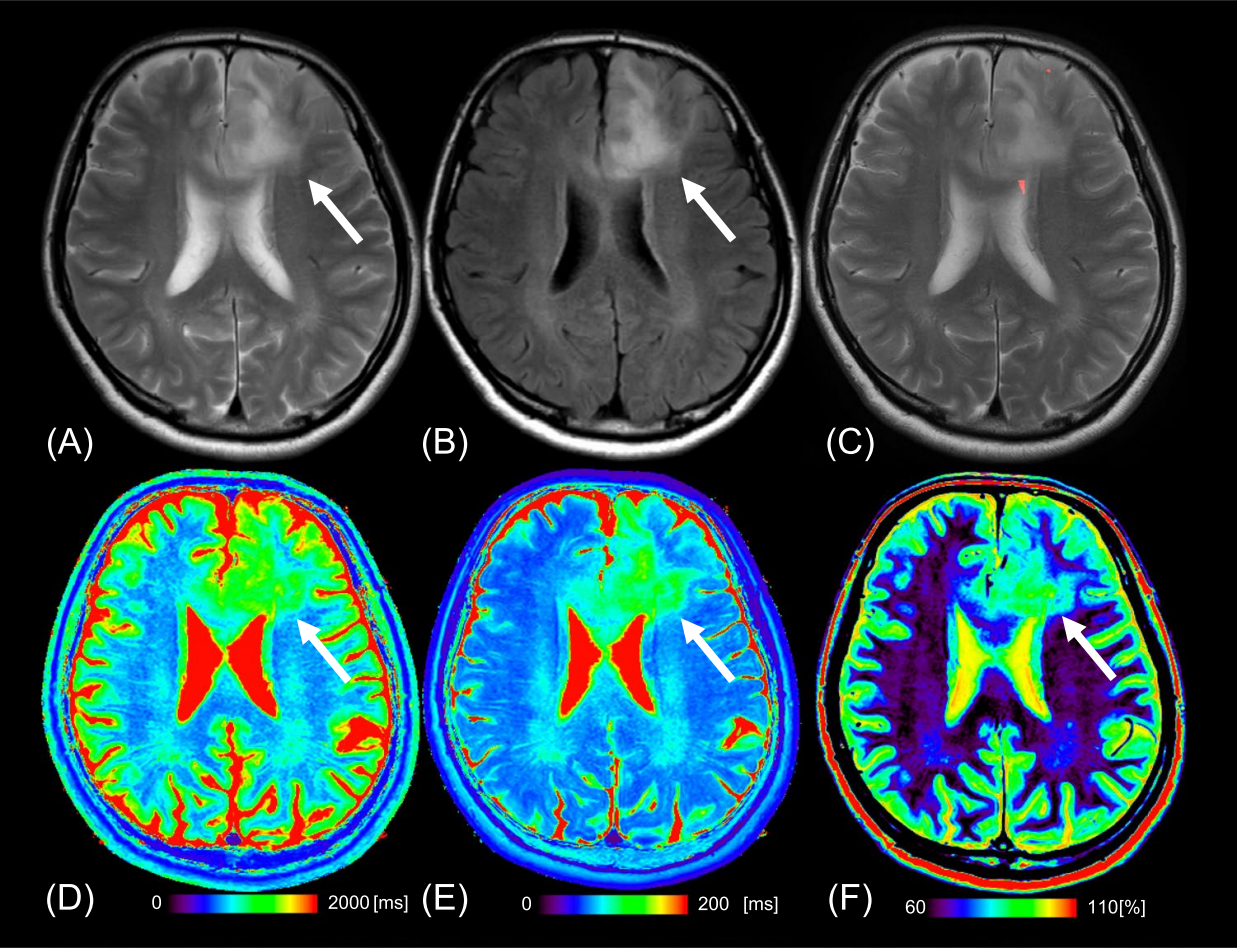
Images from a 54-year-old man with oligodendroglioma, IDH-mutant and 1p/19q-codeleted (WHO Grade 2). a T2WI shows a heterogeneous, poorly circumscribed mass in the bilateral frontal lobes (arrow). b FLAIR shows no signal suppression in the tumor, indicating no T2-FLAIR mismatch sign (arrow). c Our artificial intelligence does not correctly show this T2-FLAIR mismatch lesion. T1 (d), T2 (e), and relaxation time and proton density (f) maps derived from SyMRI show mild T1 (1783 ms*) and T2 (121 ms*) relaxation time prolongations and mildly increased PD (81.7%*) (arrows) in the tumor. Asterisks (*) beside the values in the caption indicate that each value is expressed as the mean
